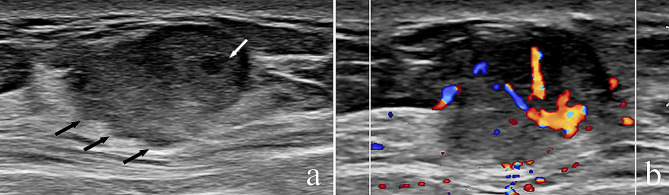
Ultrasonography of a PMBC tumor without ALNM. (a) Ultrasonogram shows a tumor with microlobulated margin (black arrow), complex cystic (white arrow) and solid echo. (b) Color Doppler flow imaging shows high level of blood flow in the tumor
